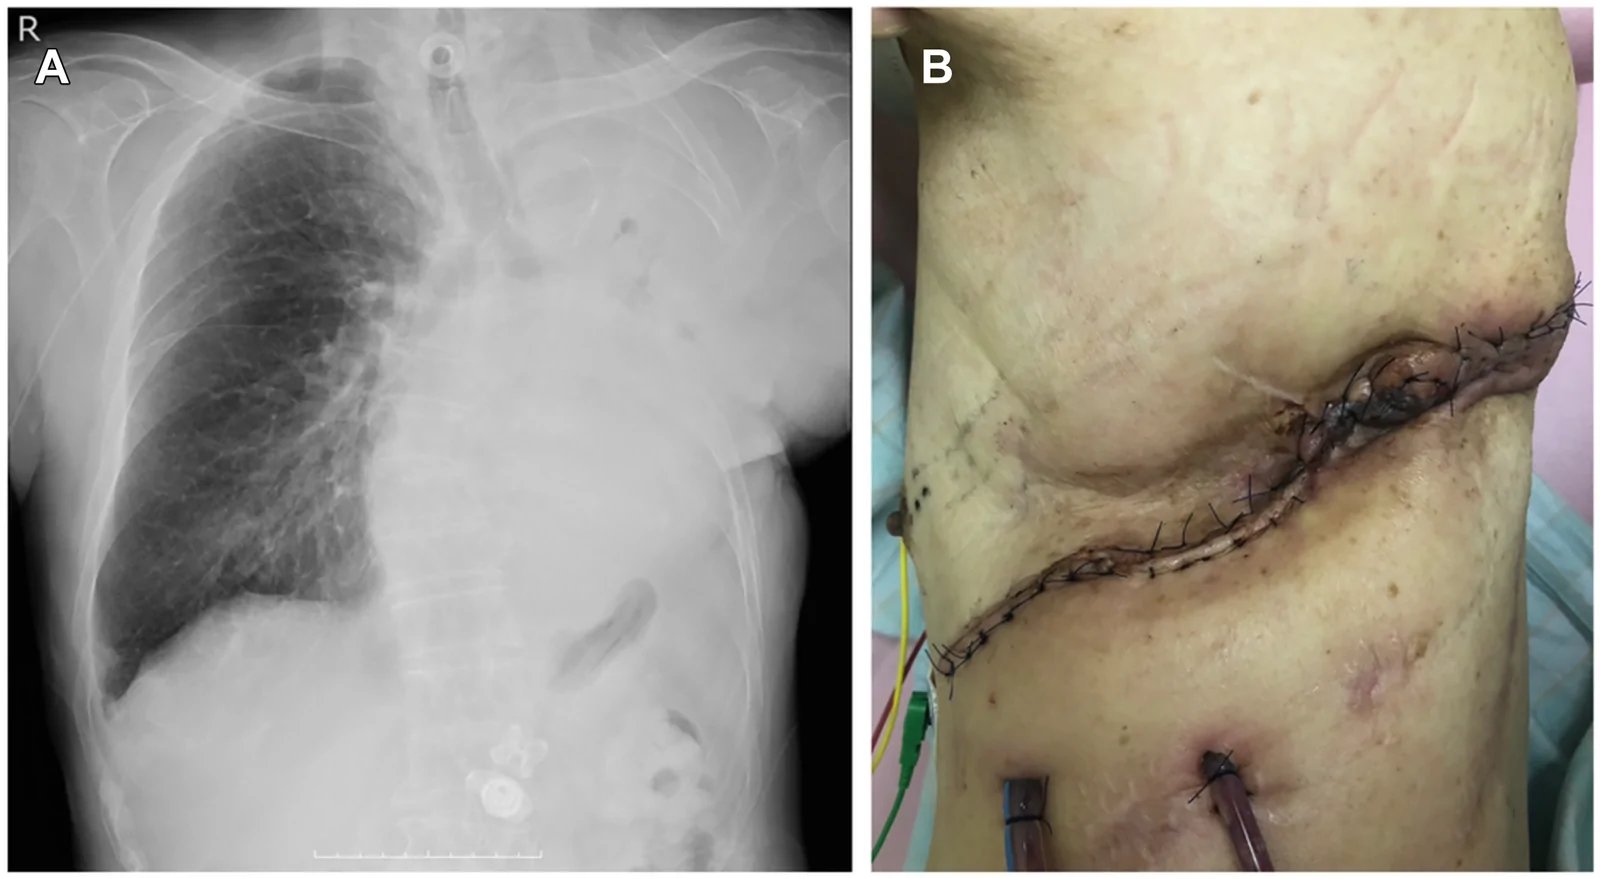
Postthoracoplasty chest radiograph (A) and (B) appearance confirming cavity obliteration.
